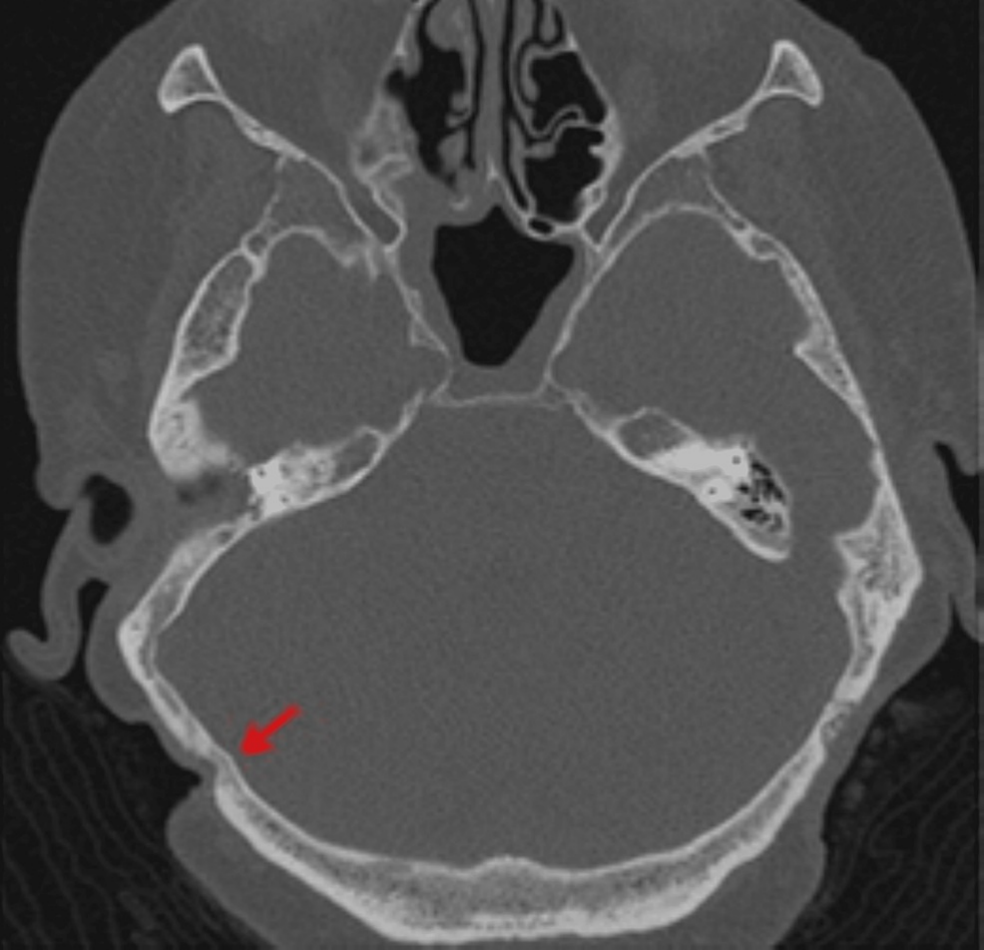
CT scan showing bony changes in the right occipital bone at the previous BAHA site one year after extrusion on November 23, 2013 BAHA: Bone-anchored hearing aids.
